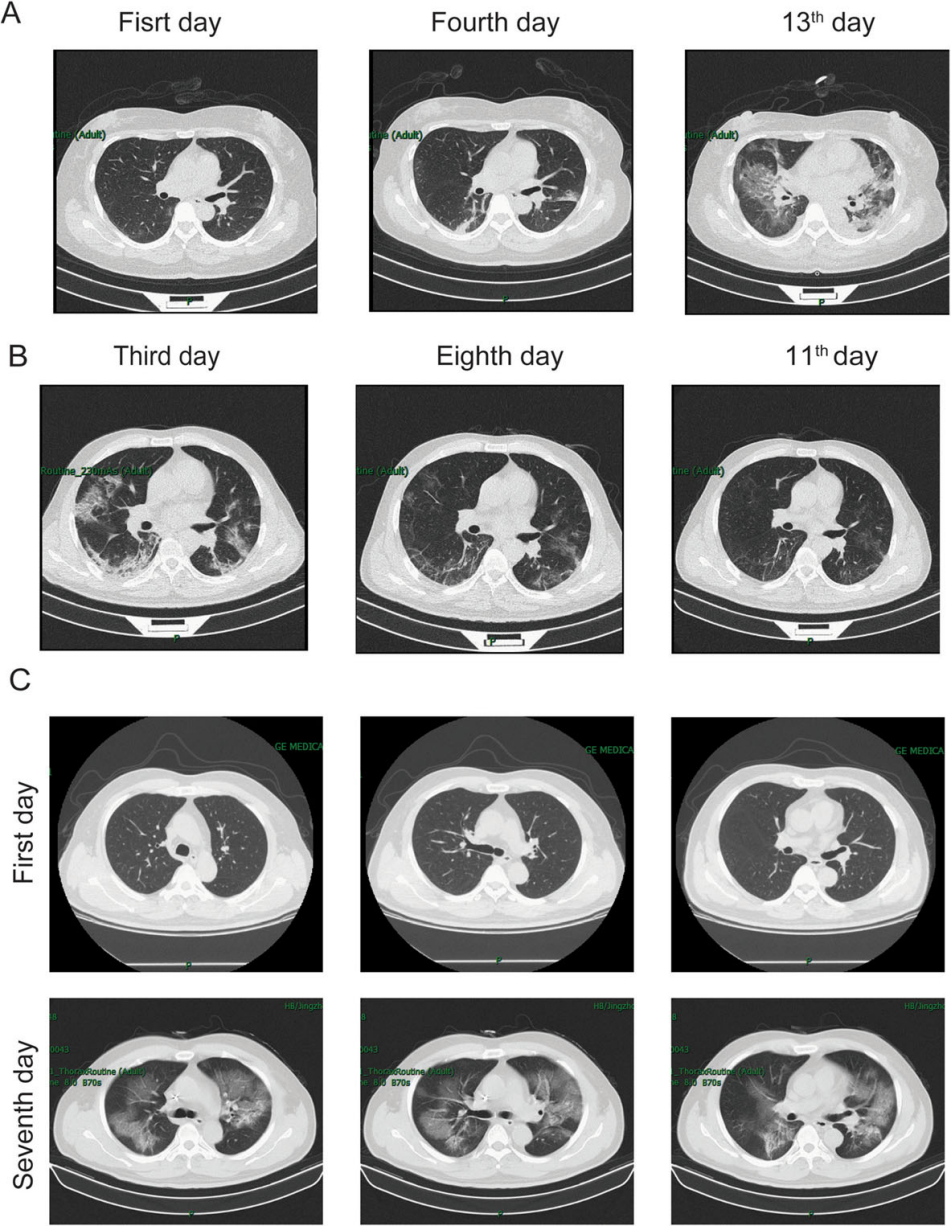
Chest CT images of patients with COVID-19. a Chest CT images of case 1 on different days after admission. b Chest CT images of case 2 on different days after admission. c Chest images on different days of a patient who died of respiratory failure
